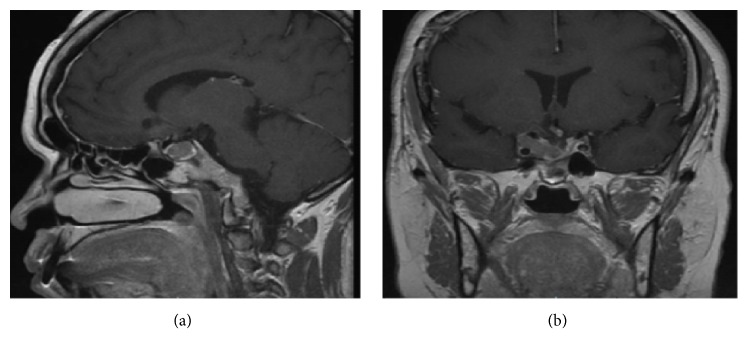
Sagittal and coronal MRI T1 sequences with gadolinium. Residual tumoral tissue only located intrasellar and right parasellar after second surgery.
